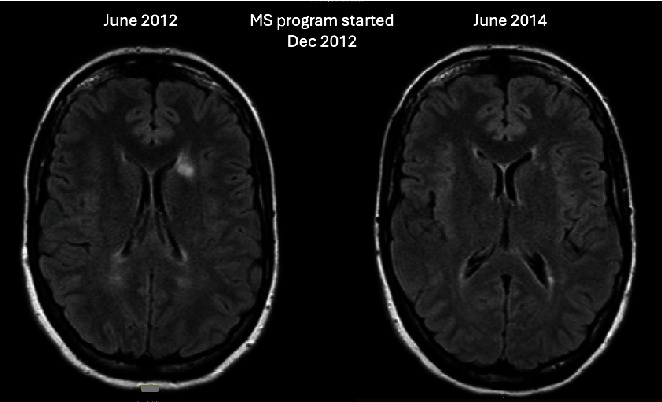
Resolution of white matter lesions in one of the designated pwMS who could not be scanned using 3-T MRI due to claustrophobia. MRIs in 2012 and 2014 were done in a hospital using sedation under clinical supervision by a neurologist.
